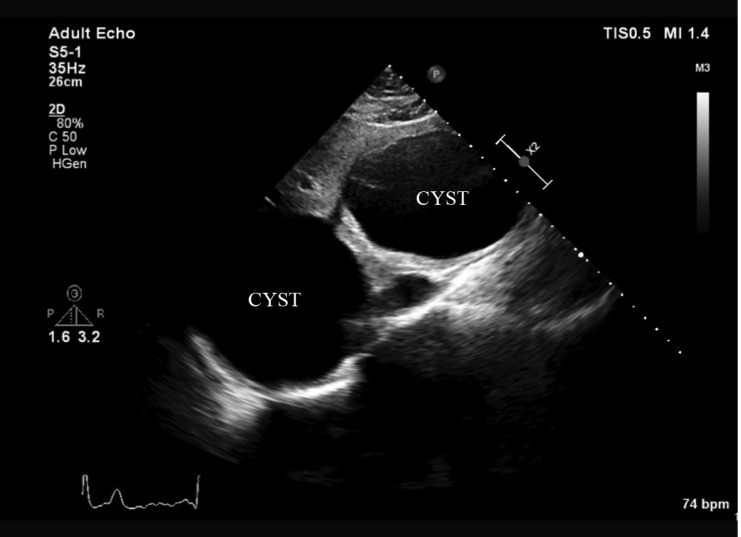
Modified substernal echocardiography view, showing 2 cysts in the liver.
